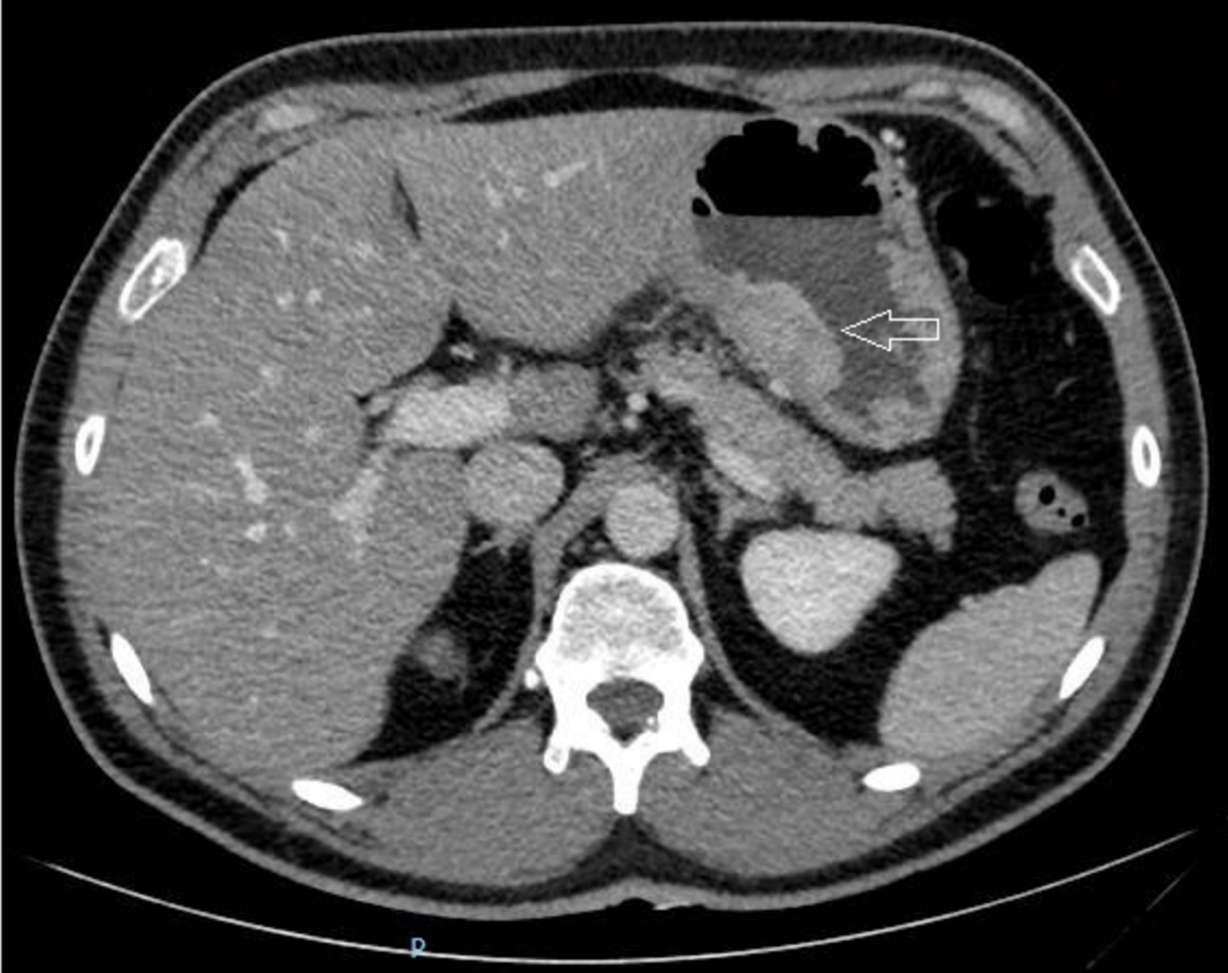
Abdominal computed tomography (CT) scan. Intraparietal nodule (2 x 3 cm) of the gastric lesser curvature (arrow).
